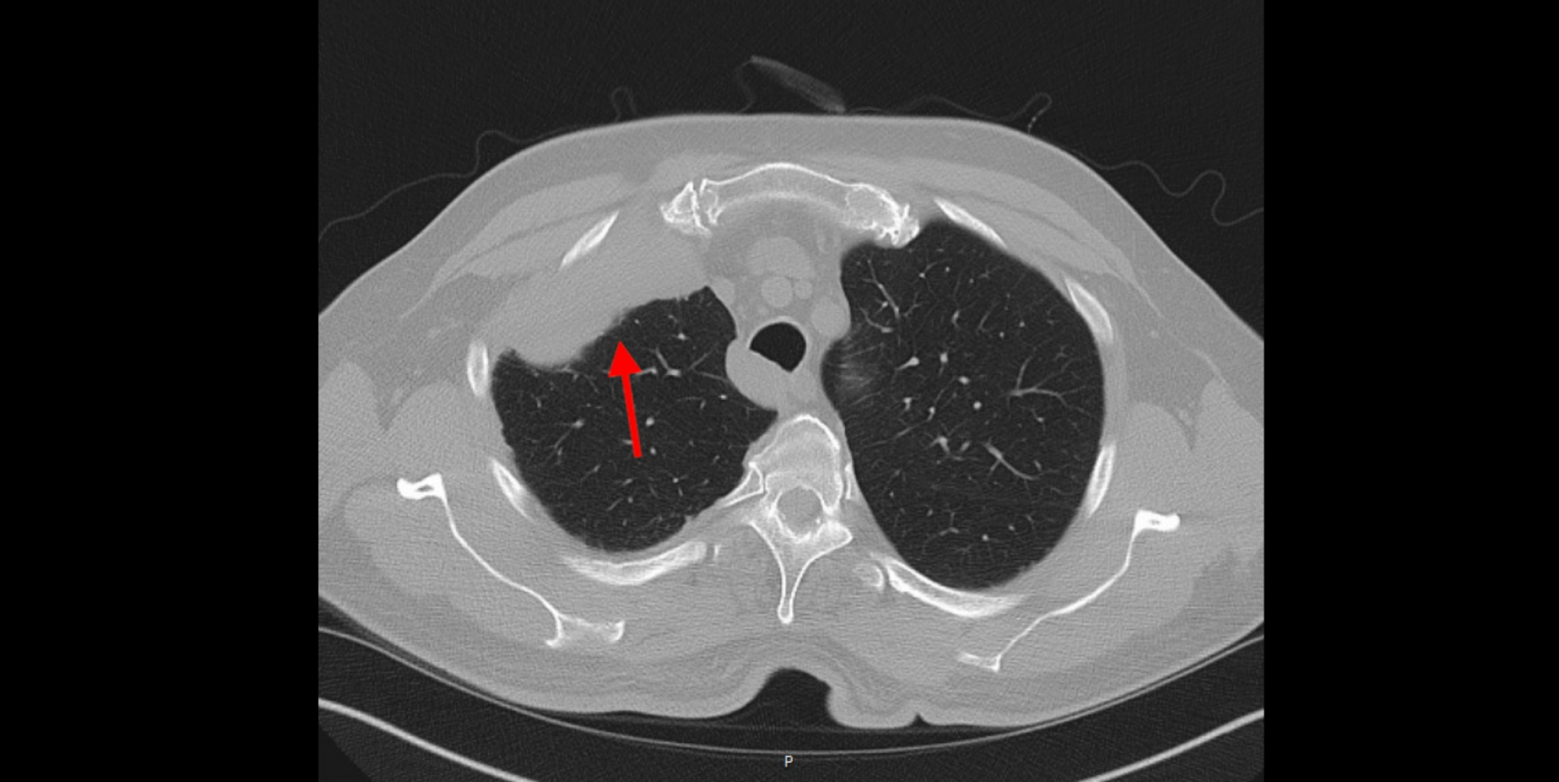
Transverse cross-section of CT Chest. Anterior pleural mass of the right upper lobe (red arrow).
